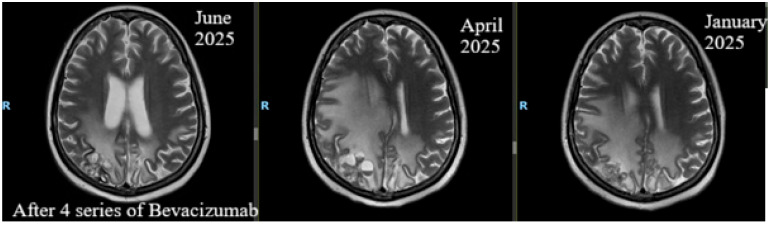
July 2025. Brain perfusion MRI after 4 cycles of treatment showing favorable overall appearance, evidenced by improved intra-axial post-therapeutic changes.
